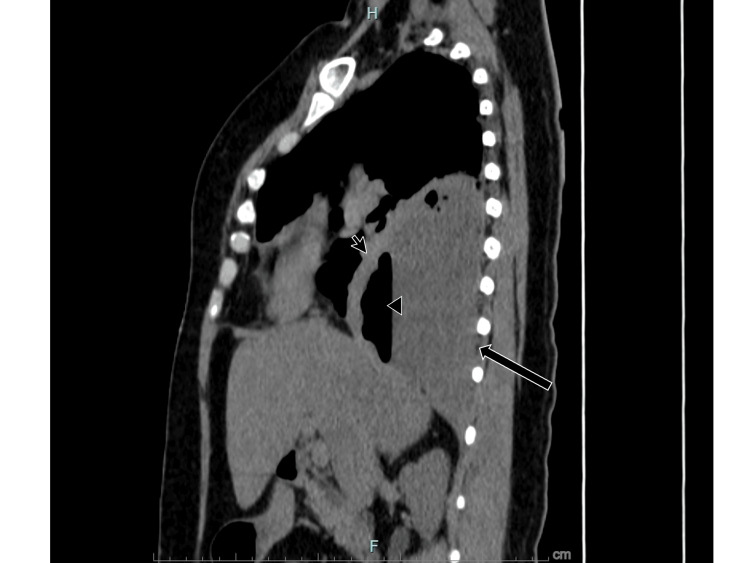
CT pulmonary sagittal view. Black arrow: Heterogeneous hypodense material in the pleural space. Small arrow: Pleural thickening. Arrowhead: Hydro-air level.
